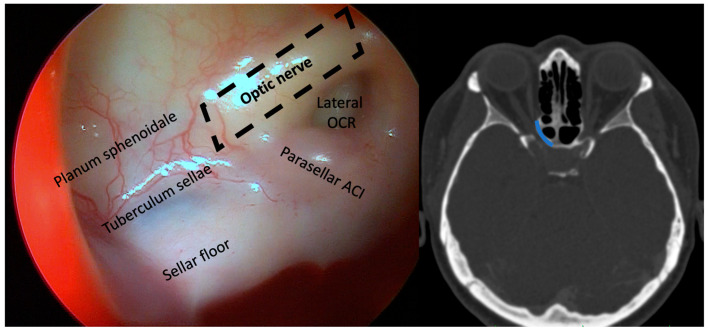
Left: sphenoid sinus view of the left ON and parasellar internal carotid artery. The dotted line outlines the optic canal. Right: axial CT imaging in bone window. The line highlights the extent of optic canal decompression.
